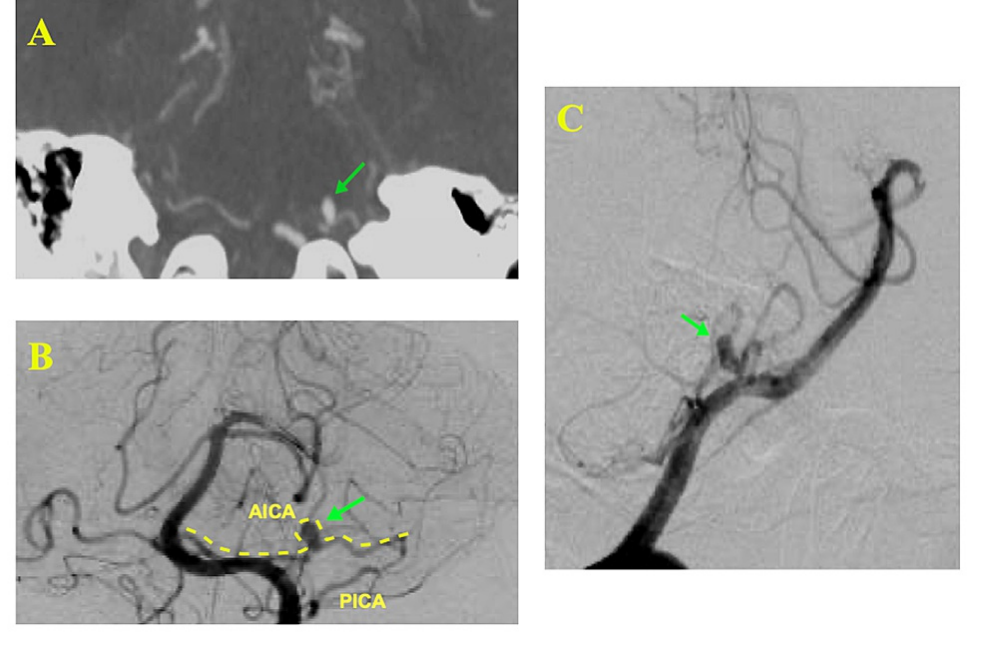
CT angiogram and diagnostic cerebral angiogram images show (A) AICA aneurysm in coronal view (arrow), (B) AICA aneurysm in AP view (arrow), (C) AICA aneurysm in lateral view (arrow). AICA: anterior inferior cerebellar artery; PICA: posterior inferior cerebellar artery; AP: anteroposterior
